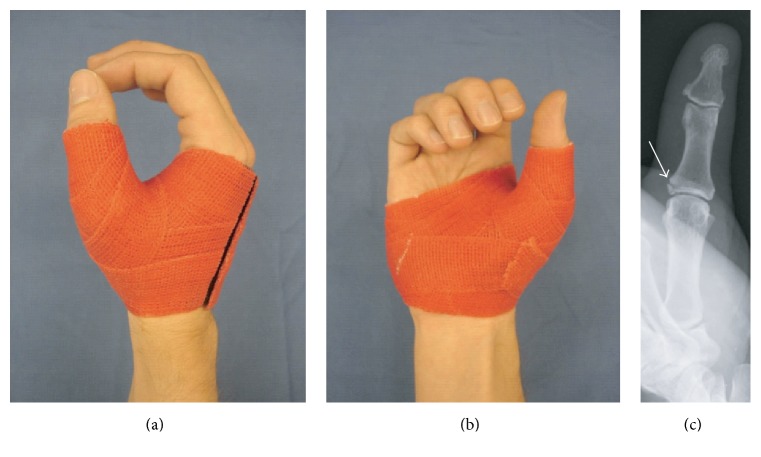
Thumb orthoses: (a) dorsoradial and (b) palmar view and (c) case sample: X-ray at 6 weeks after wearing orthoses following bony ulnar collateral ligament traumatic lesion (DASH analysis of case series: *n* = 11; score-mean = 29.9 ± 1.73 SD; DASH follow-up interval (weeks): 9.5 ± 2.01 SD).
